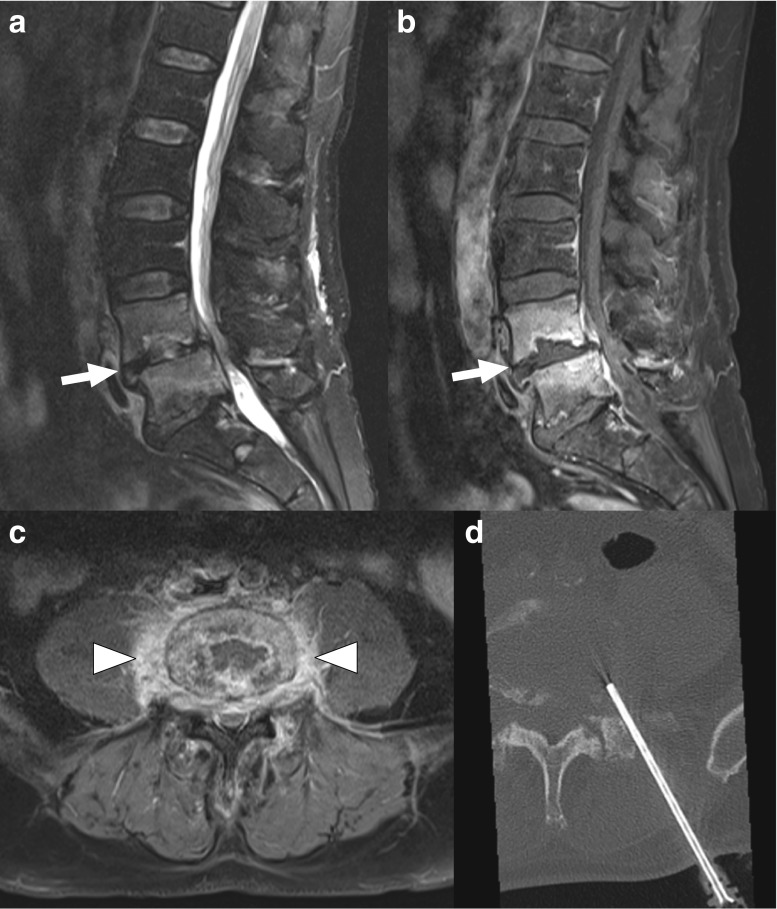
A 67-year-old man with MRI findings typical of spondylodiscitis. Sagittal fat-suppressed T2-weighted (a) and gadolinium-enhanced T1-weighed images (b) show involvement of the L4 and L5 vertebrae (*arrows*), and also increased T2 signal of the L4-L5 disc (a). Axial gadolinium-enhanced T1-weighted image (c) shows a paravertebral phlegmon (*arrowheads*). CT-guided biopsy was performed (d), with positive cultures for *Staphylococcus warneri*
